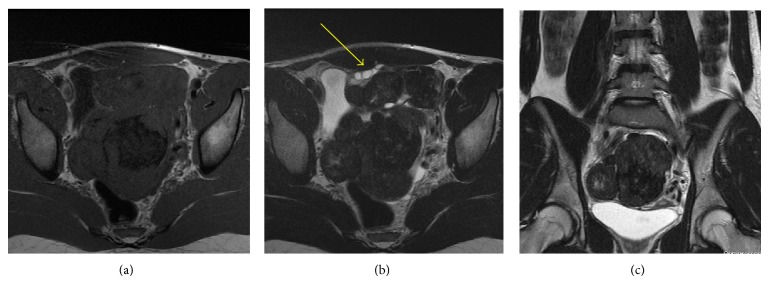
MRI imaging. (a) Axial T1-W image through the pelvis shows 1 contiguous or multiple soft tissue pelvic masses demonstrating isointense T1 signal when compared with normal muscle and a hypointense central component that represents the fibrous portion of the mass. (b) Axial T2-W image demonstrates multiple normal ovarian follicles within the anterior portion of the mass (*yellow arrow*), localizing the pelvic mass to the ovary. (c) Coronal T2-W image demonstrates heterogeneous solid masses predominately intermediate to low intensity T2 signal.
